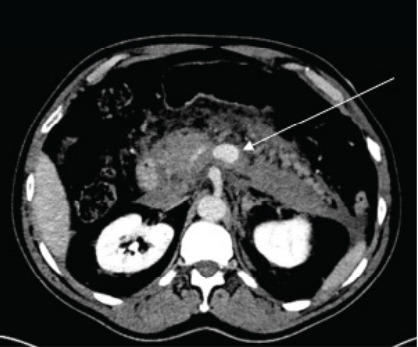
Computed tomography angiogram abdomen and pelvis during the delayed venous phase demonstrating a large amount of hyperdense material surrounding the pancreas extending into the left anterior perirenal space. A focal contrast blush is visible just anterior to the celiac artery (arrow).
